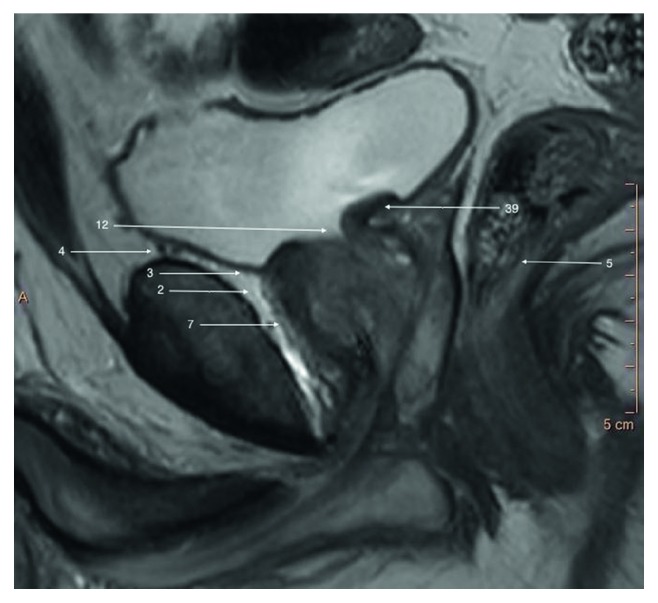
T2-weighted sagittal image of a prostate gland and surrounding structures.
